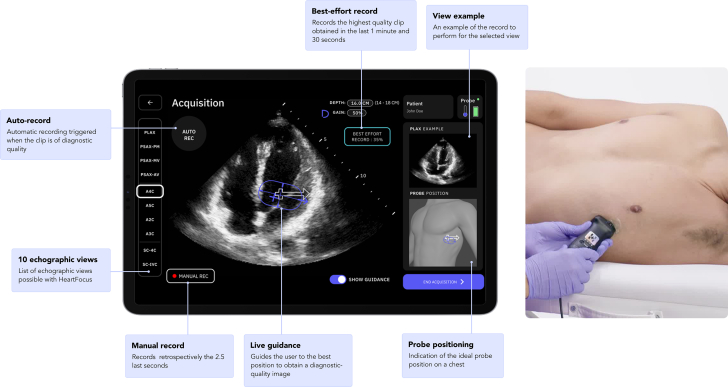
Artificial Intelligence-Based Software User Interface and Setup for Echocardiographic Acquisition An example of the studied application screen (left) showing the actual view in real-time with the Live Guidance items (middle of the screen), the recording features (auto record, best-effort record, manual record), the 10 echocardiographic views (left of the screen), the view example and the probe positioning (right of the screen), and the setup for the apical 4-chamber view acquisition. A2C = apical 2-chamber; A3C = apical 3-chamber; A4C = apical 4-chamber; A5C = apical 5-chamber; PLAX = parasternal long-axis; PSAX-AV = parasternal short-axis at the aortic valve; PSAX-MV = parasternal short-axis at the mitral valve; PSAX-PM = parasternal short-axis at the papillary muscle; SC-4C = subcostal 4-chamber; SC-IVC = subcostal inferior vena cava.
